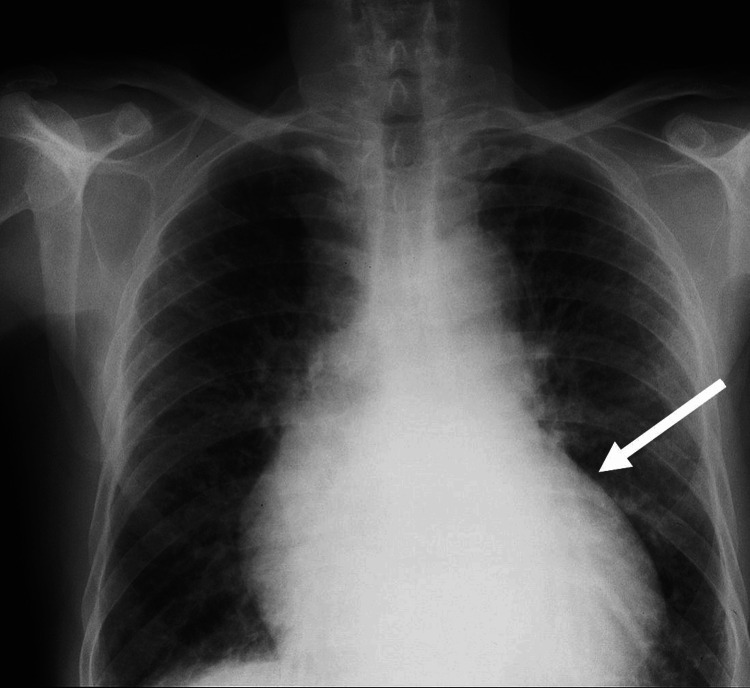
Initial chest X-ray showing cardiomegaly with increased overall dimensions of the heart.
